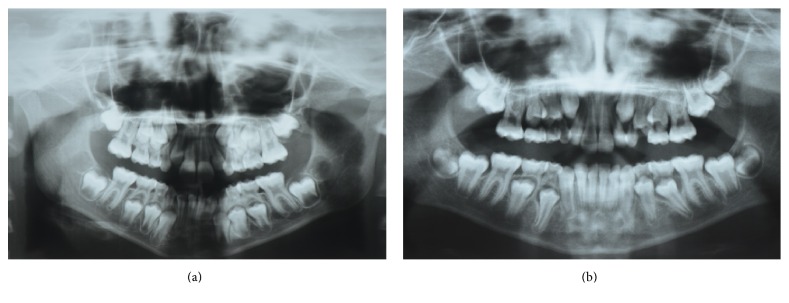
(a) Panoramic radiograph of the patient aged 7 years and 3 months and (b) 9 years and 4 months with no evidence of supernumerary teeth.
